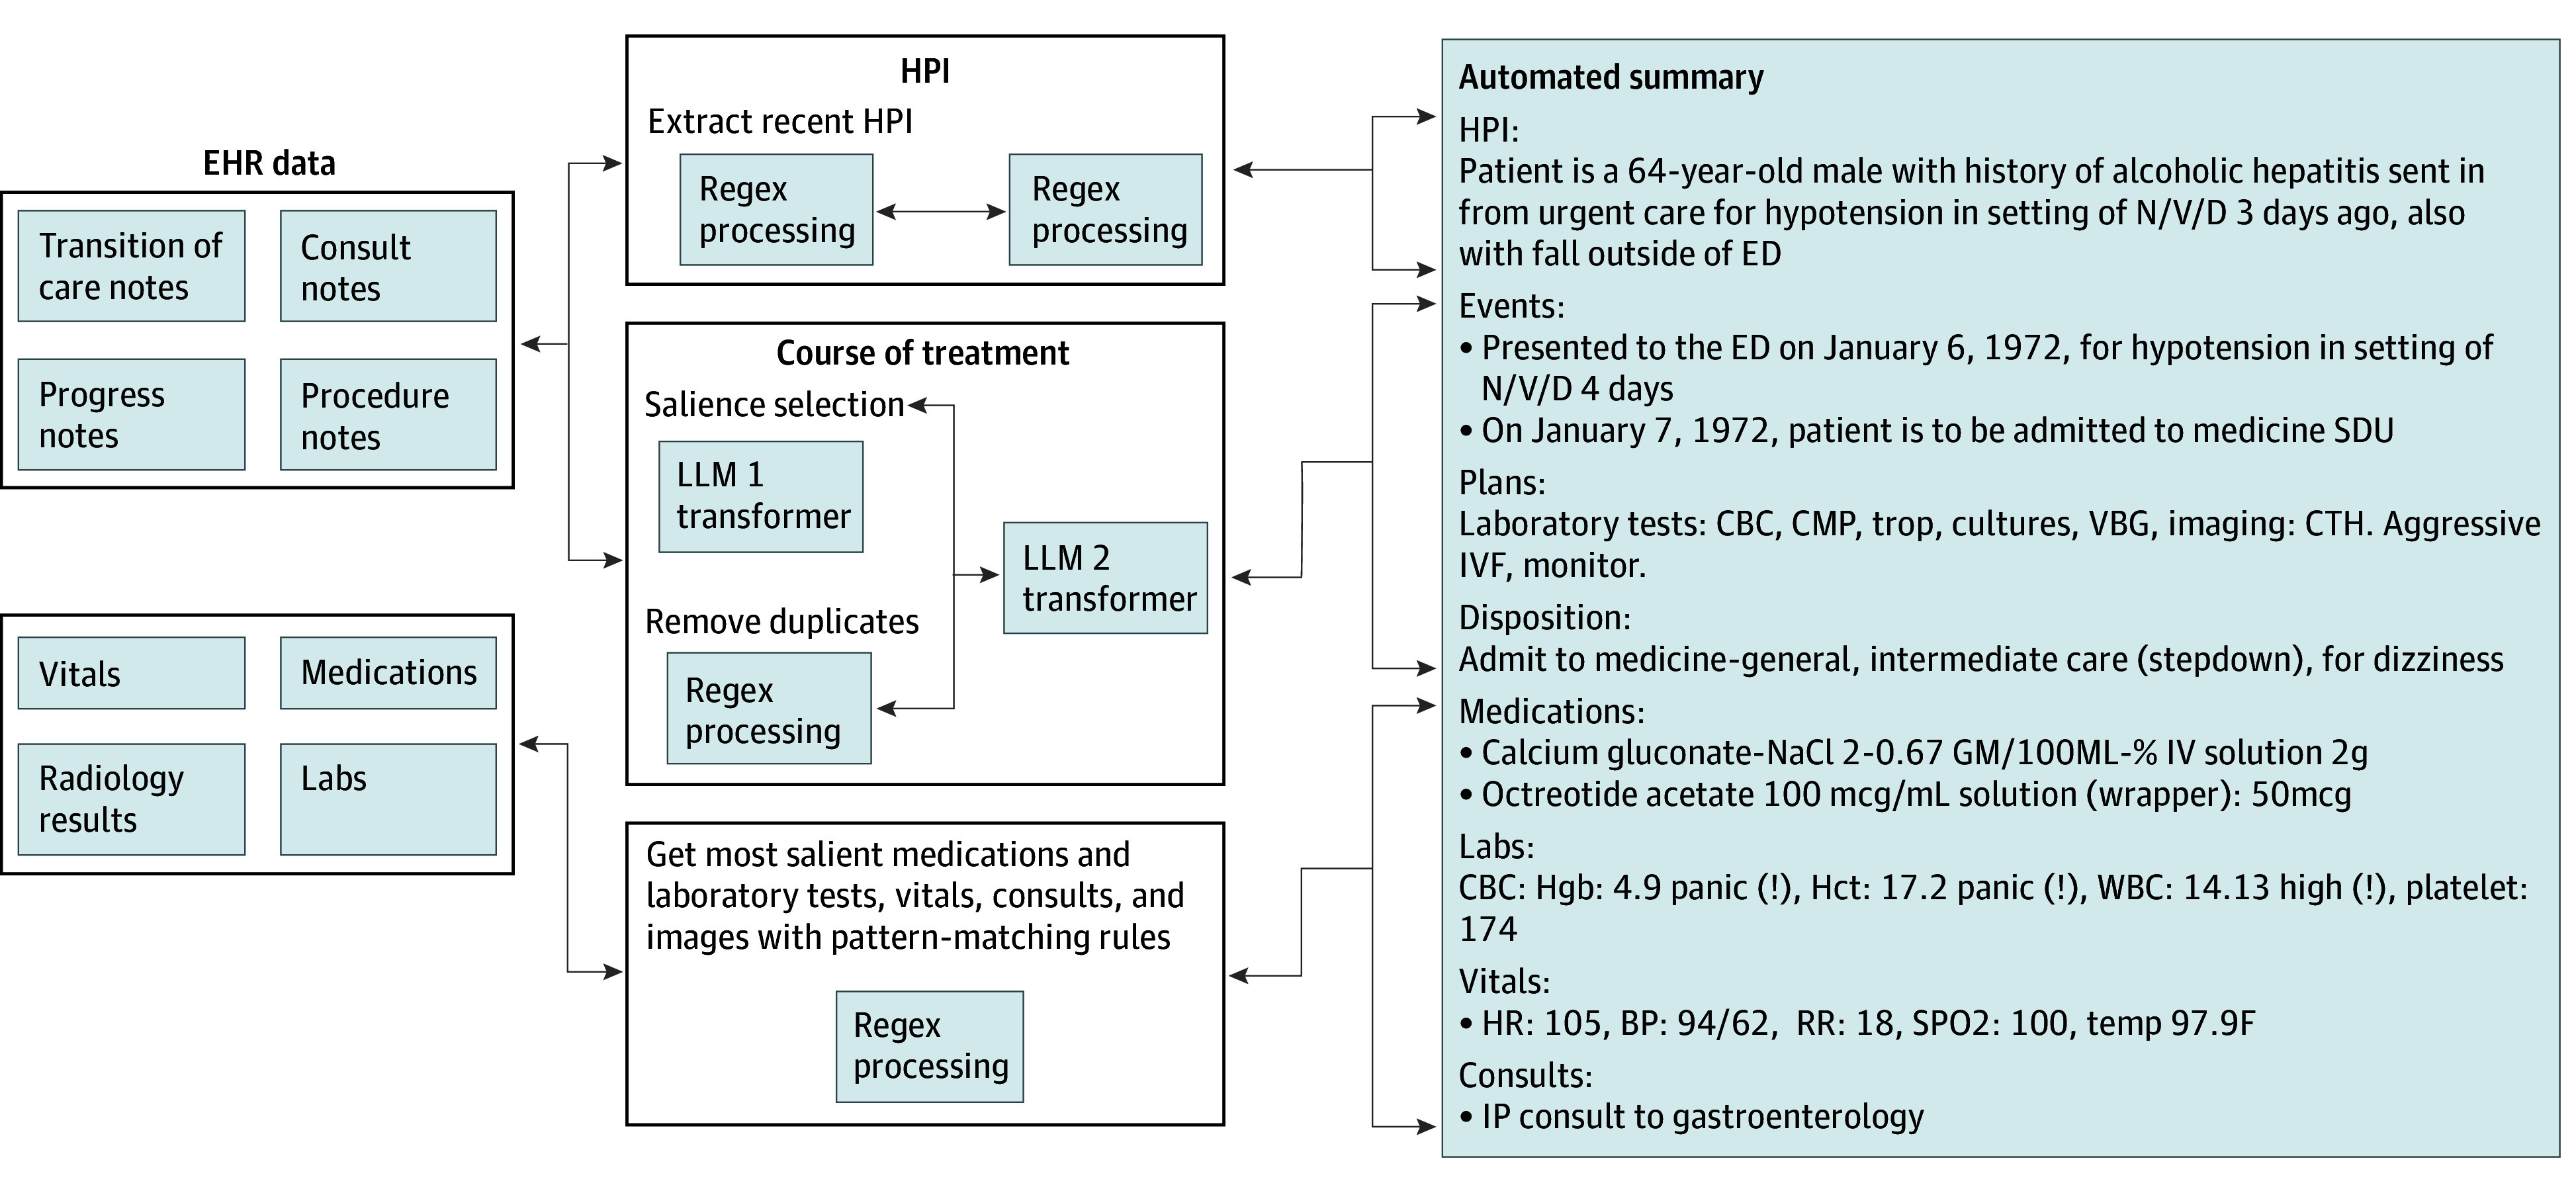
Data Flow of Generating Emergency Department (ED) Handoff Summary CBC indicates complete blood count; CMP, comprehensive metabolic panel; CTH, computed tomography of the head; EHR, electronic health record; Hct, hematocrit; Hgb, hemoglobin; HPI, history of present illness; HR, heart rate; IP, inpatient; IVF, intravenous fluid; N/V/D, nausea, vomiting, and diarrhea; RR, respiratory rate; SDU, step down unit; SPO2, peripheral capillary oxygen saturation; WBC, white blood cell; WBG, whole blood glucose.
